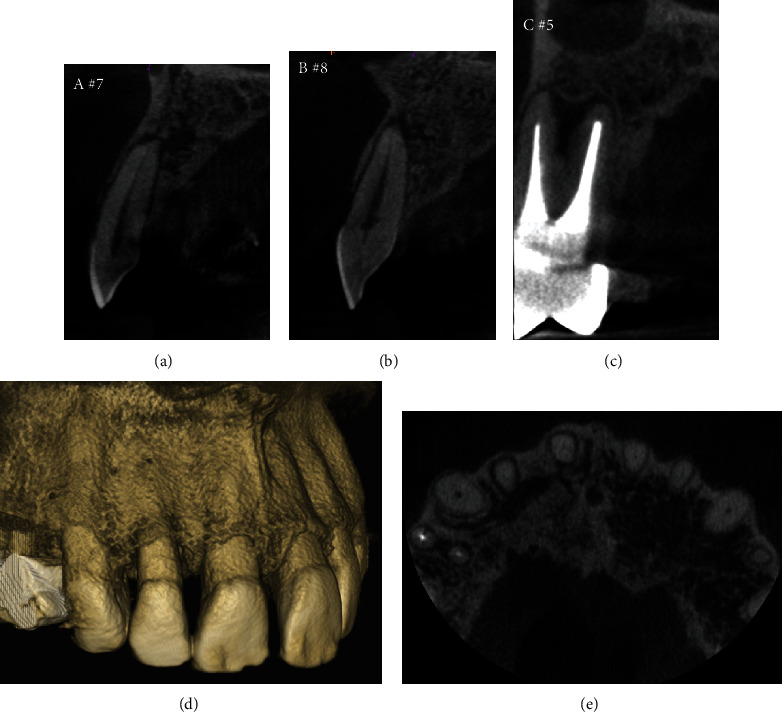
Postoperative CBCT images. CBCT images of the maxillary right central and lateral incisors after a three-year period from the retreatment of only the first premolar; the hypodense regions had almost disappeared (a–c). Volume rendering image after three years showed that the buccal cortical bone had clearly recovered (d). Axial image of maxillary front to premolar area after three years shows the progress of osteoanagenesis in the palatal area (e).
